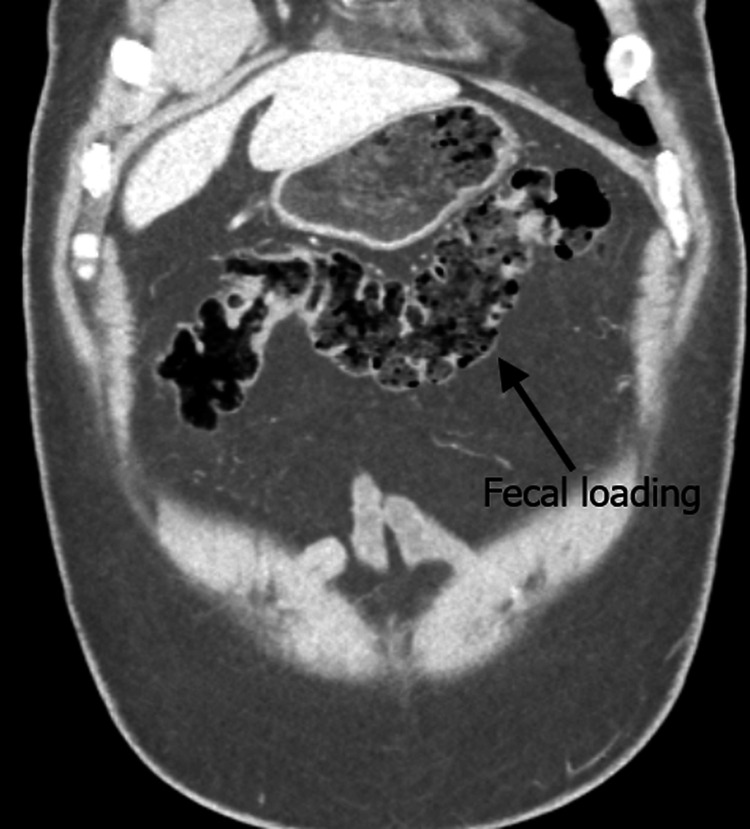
Axial CT scan demonstrating fecal loading in the transverse colon
